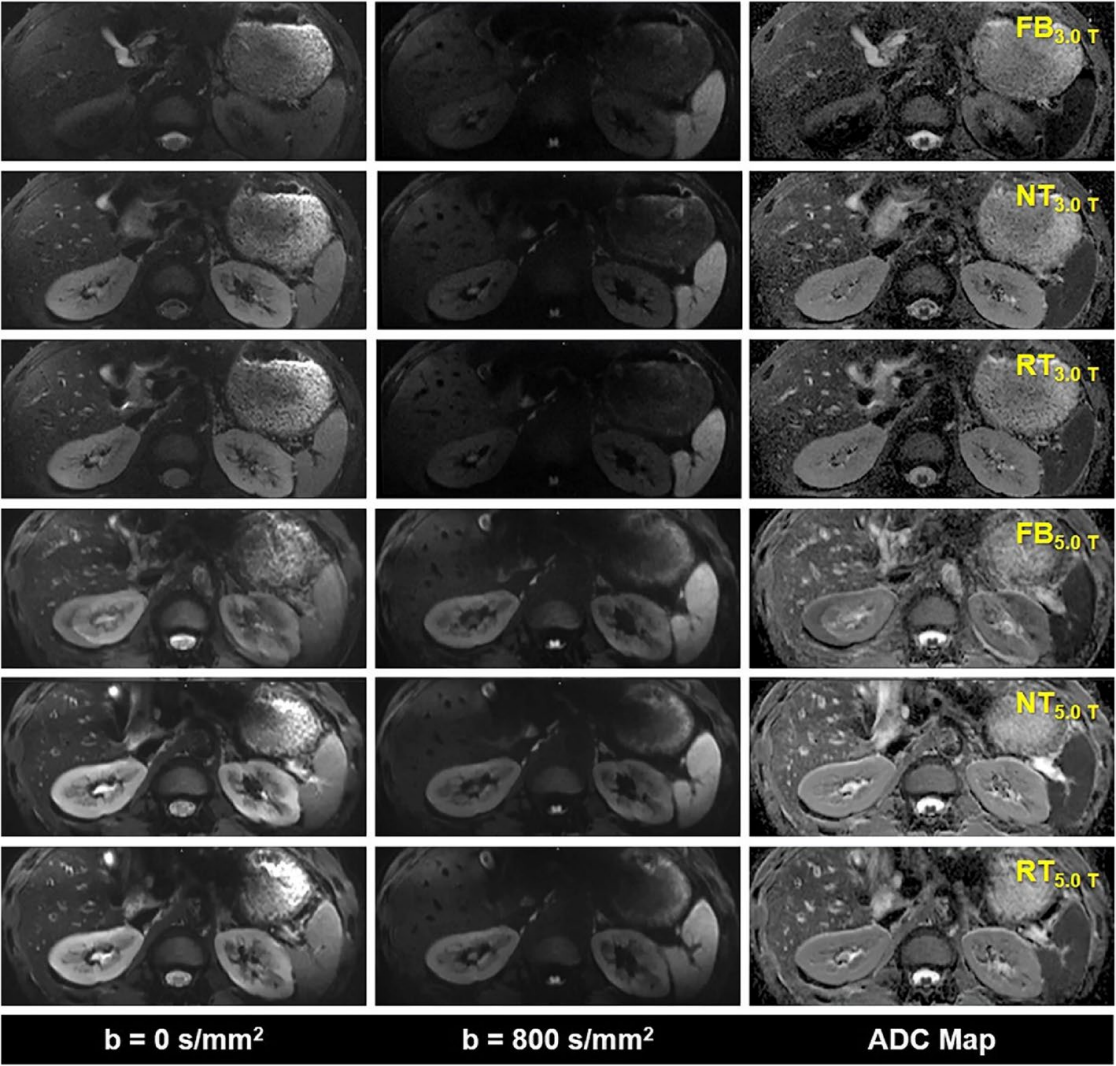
Representative MR images of a 25-year-old man weighing 65.0 kg and with a BMI of 20.5. The DWI images obtained by six acquisition approaches are listed in different rows. The DWI images of *b* = 0 s/mm^2^, *b* = 800 s/mm^2^, and ADC parametric maps are displayed in different columns
